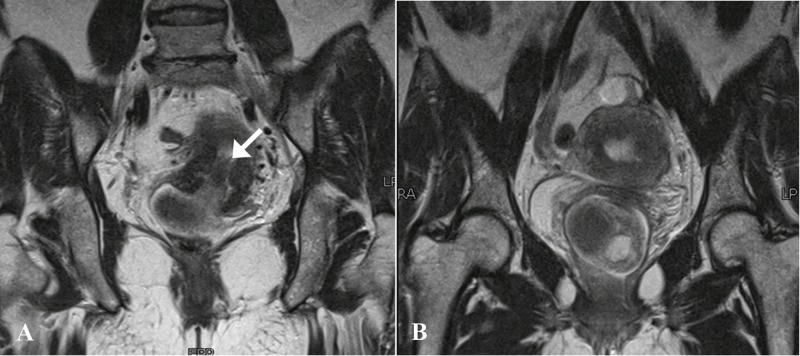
Magnetic resonance images - Coronal T2-weighted sequences. A) A T2-hypointense stalk was seen extending inferiorly from the endometrium and cervix (arrow). B) The stalk was connected to the polypoidal lesion with cystic spaces appreciated within the vagina.
